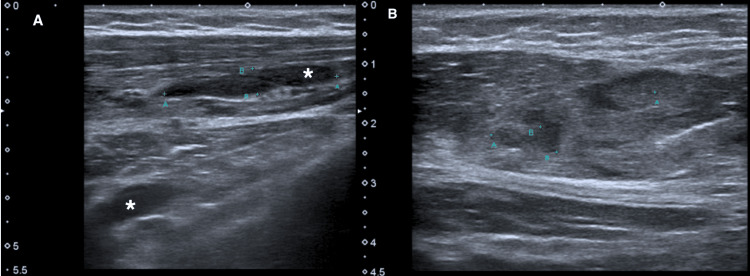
Ultrasound of the pectoral muscles, different views, showing multiple areas of liquid collection (asterisk) on panel A and muscle tissue heterogeneity on panel B
